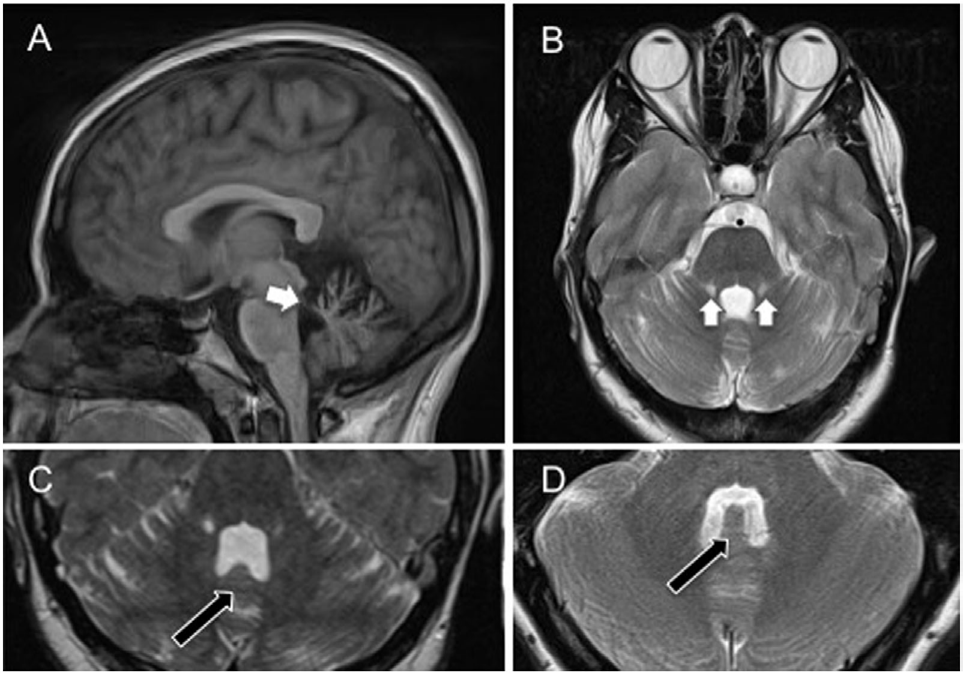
(A) Sagittal cross-sectional T1-weighted magnetic resonance image showing atrophy of the superior cerebellar peduncles, and anterior aspects of the cerebellum (white arrow) involving the uvula and nodulus. (B) Coronal T2-weighted magnetic resonance image showing bilateral atrophy of the anterior cerebellum, including the nodulus and uvula, and superior cerebellar peduncles with associated high signal (gliotic) change. (C) Axial T2-weighted magnetic resonance image showing atrophy of the nodulus (black arrow) within the enlarged fourth ventricle. (D) Axial T2-weighted magnetic resonance image showing nodulus (black arrow) and fourth ventricle in a healthy age-matched control. Note the flattening of the nodulus and expansion of the fourth ventricle in (C).
